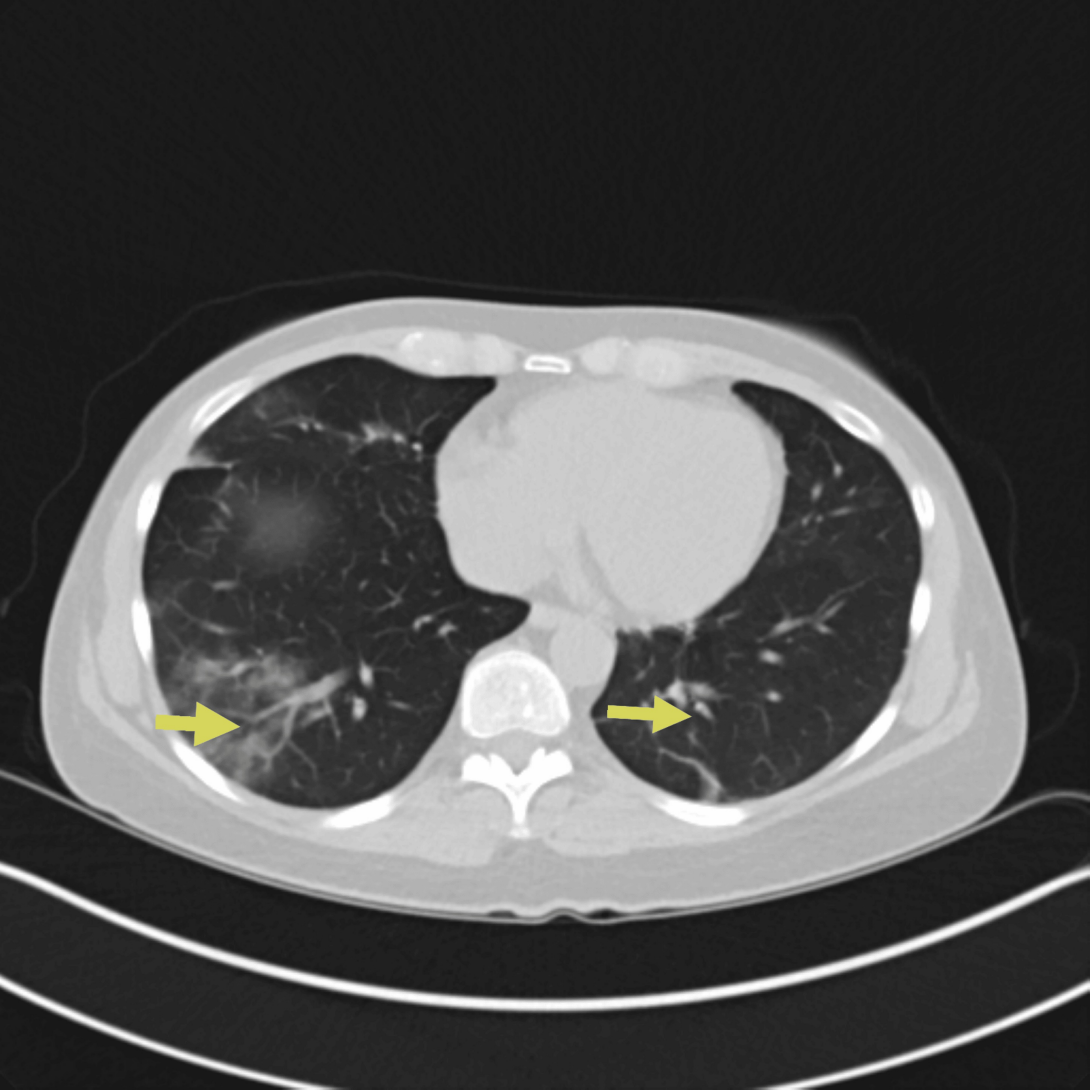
CT chest showing persistent bilateral pulmonary opacities/ground-glass opacities (yellow arrows) The CT chest findings improved compared to Figure 1.
